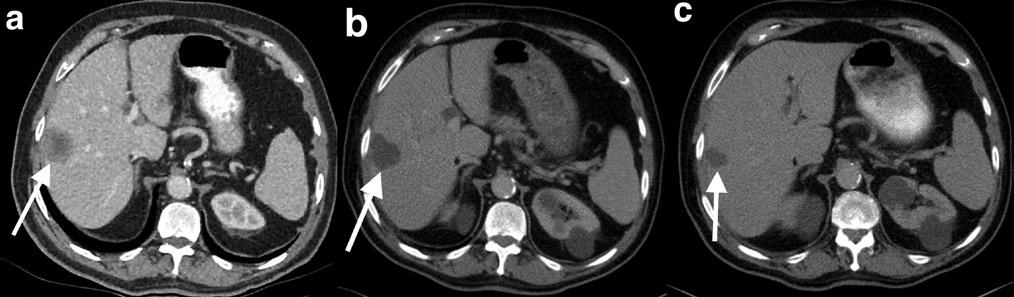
Post-treatment appearances of GIST liver metastases. Portal venous CT demonstrates a hypoattenuating segment 5/8 of the liver (a). 6 months after commencement of tyrosine kinase inhibitors, the lesion becomes more hypoattenuating but increases in size (b). Despite no change in treatment regimen, CT performed 12 months after the initial scan demonstrates reduction in size of this lesion (c). GIST, gastrointestinal stromal tumour.
